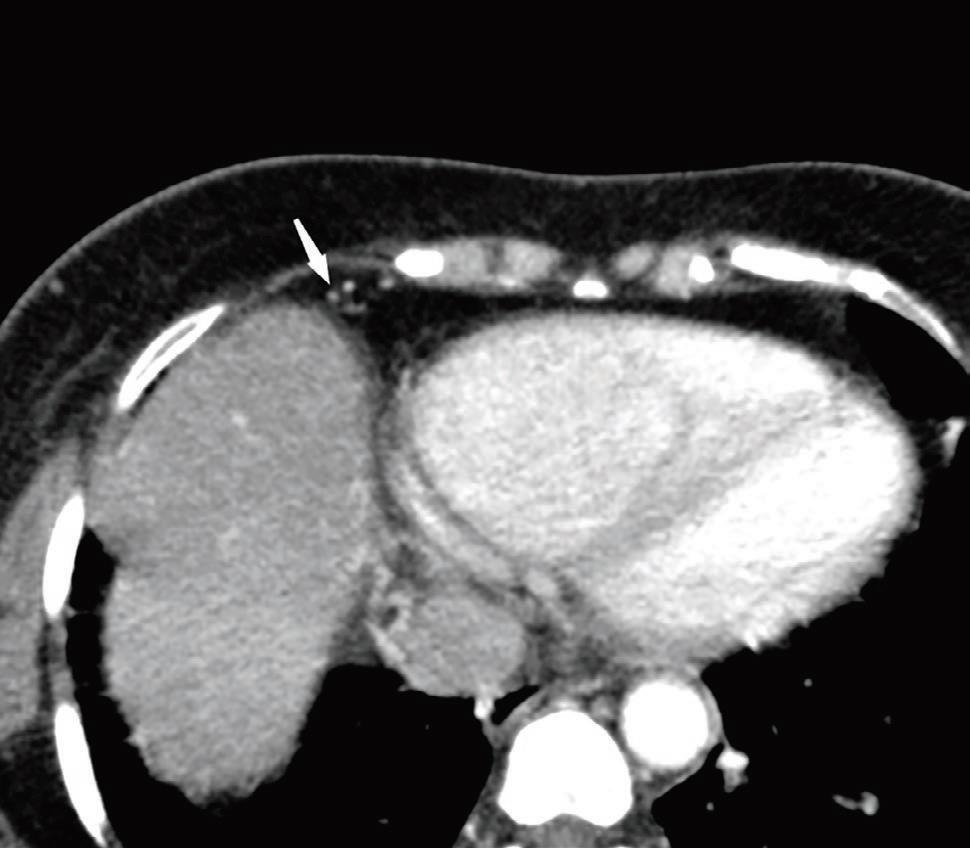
CT pulmonary angiogram phase in axial view shows collateral branch from right internal mammary artery supplying the right lung.
